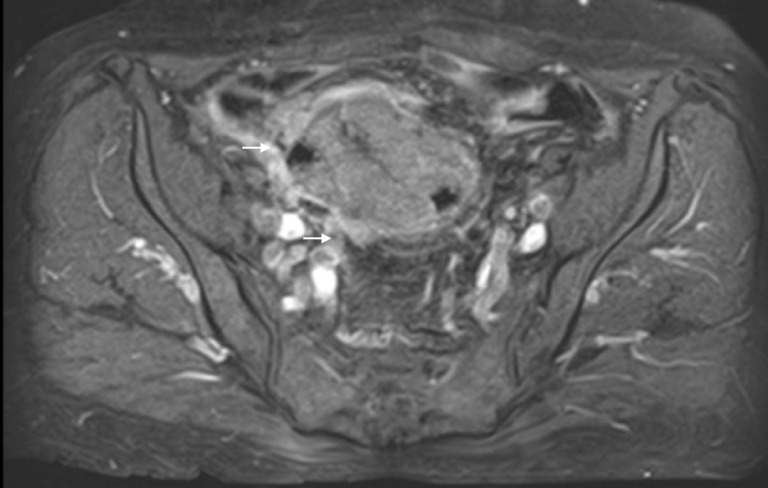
The sagittal cross section from the magnetic resonance imaging (MRI) of the abdomen-pelvis, performed in 2016, of the ovarian mass.
